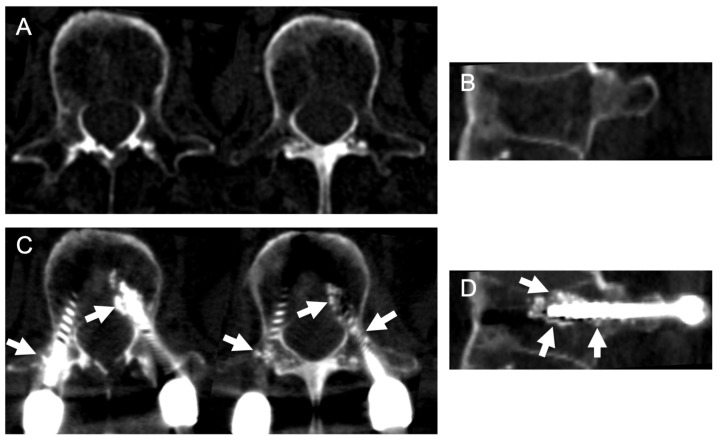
Computed tomography before (A,B) and after (C,D) surgery. Postoperative axial (C) and sagittal (D) images revealed that the HA granules surrounded the screws within the pedicle and vertebral body (Arrows).
